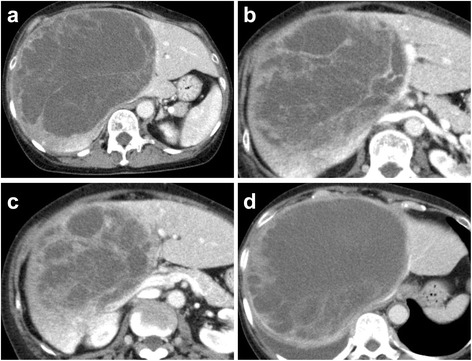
Abdominal CT revealed a huge tumor. A well-defined, low-density, heterogeneous, multilocular, cystic tumor with septa involved the right lobe and the medial segment of the liver (a). The tumor compressed the umbilical portion of the portal vein (b), the right portal vein (c), and the left hepatic vein (d)
